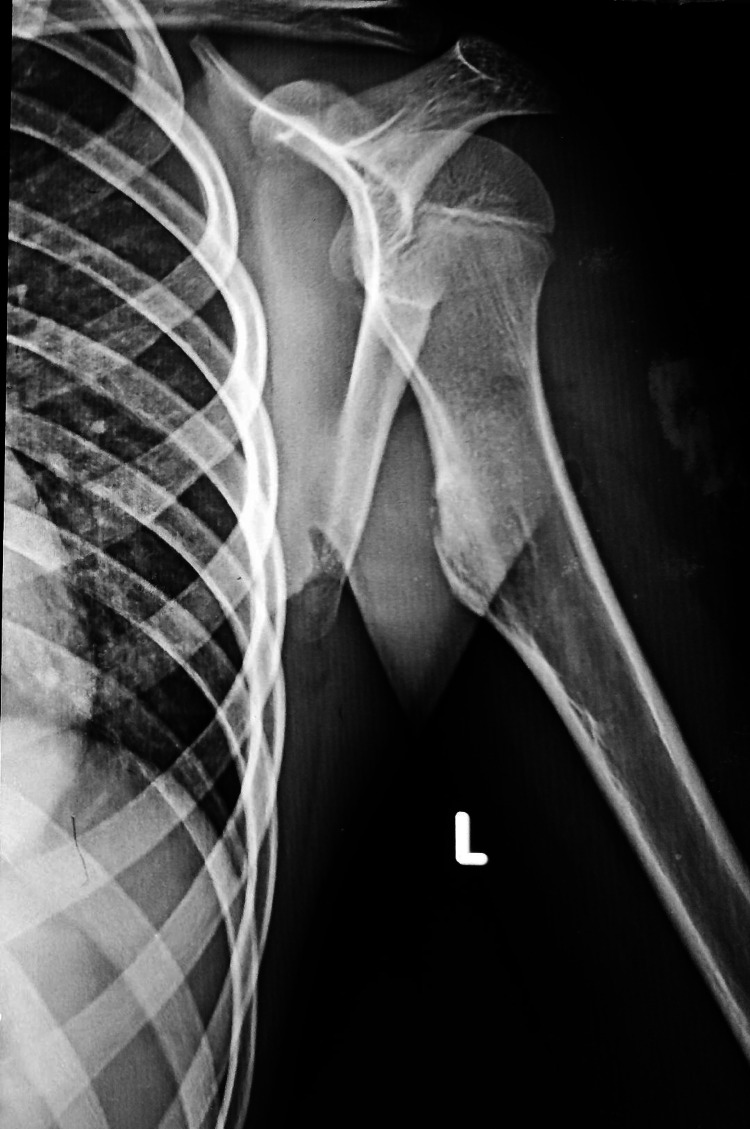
Left shoulder X-ray showing the presence of a sessile osteochondroma of the left proximal humerus at 11 years of age.
